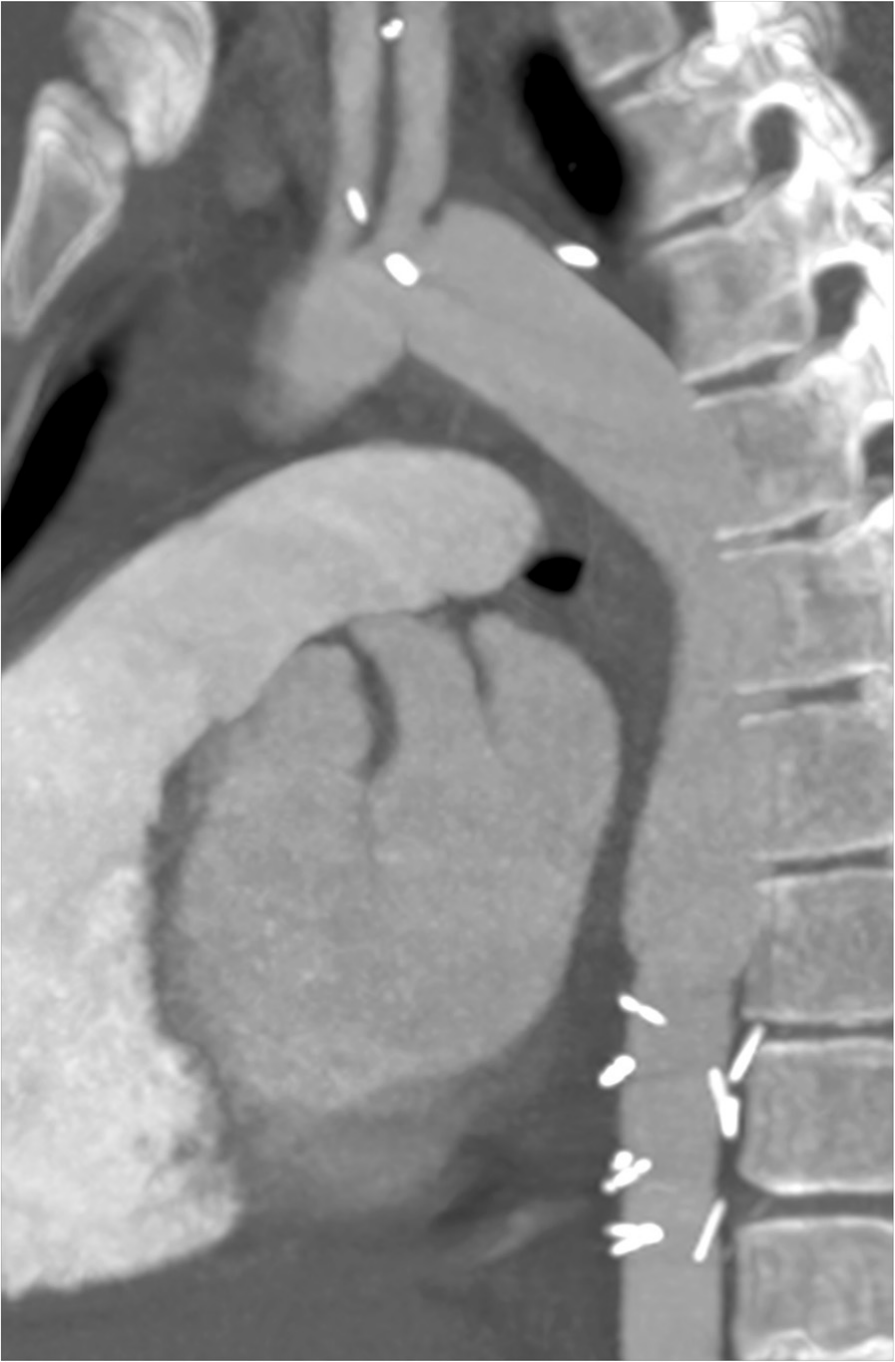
CT scan image following endograft removal and open repair, demonstrating widely patent proximal anastomosis, aortic graft, and distal anastomosis.
